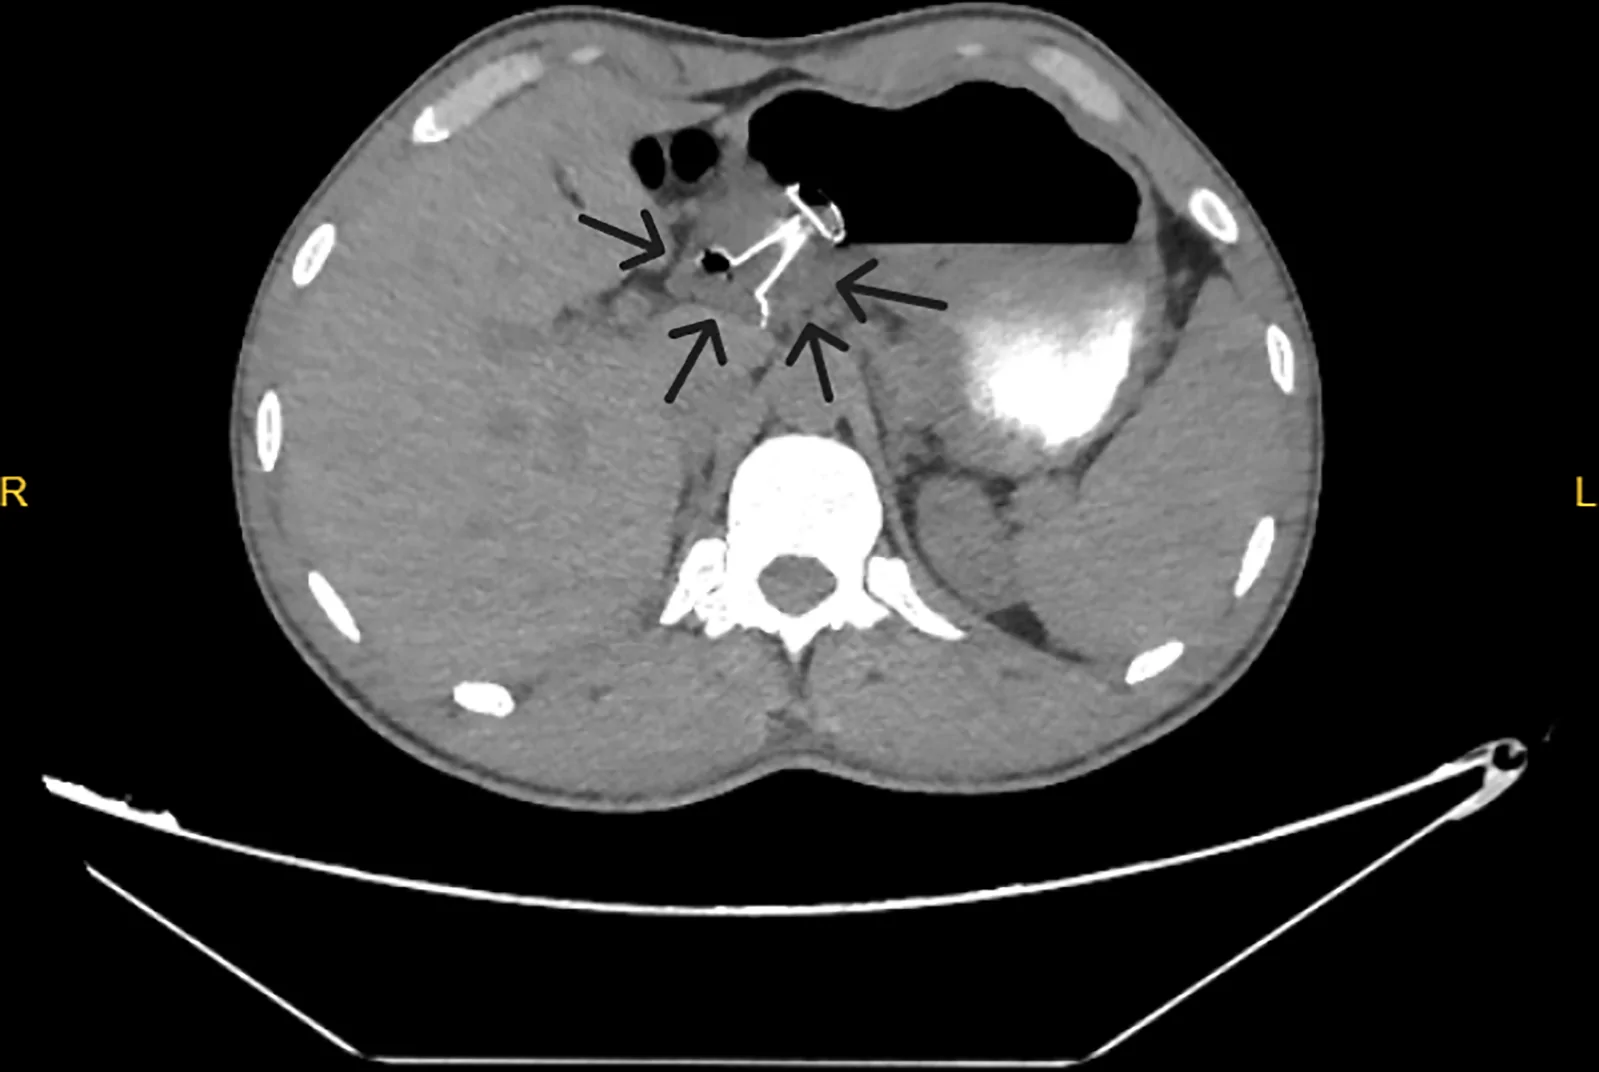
Computed tomography image showing the lumen-apposing metal stent deployed at the pylorus. Arrows indicate the precise location of the stent across the stenotic segment.
